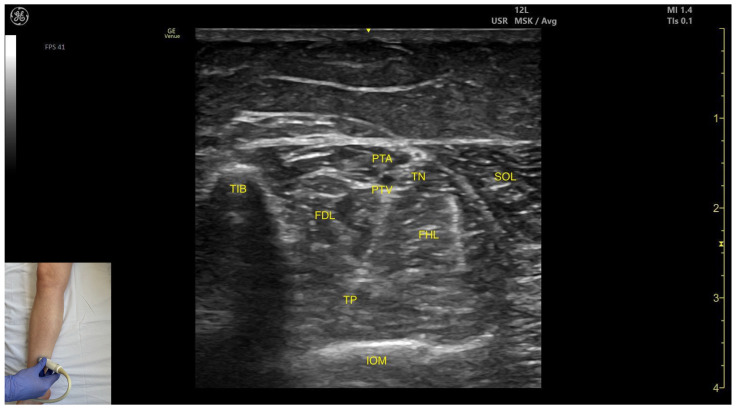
Ultrasound anatomy of the tibialis posterior—medial window: TIB—tibia; FDL—flexor digitorum longus; TP—tibialis posterior; PTA—posterior tibial artery; PTV—posterior tibial vein; TN—tibial nerve; FHL—flexor hallucis longus; IOM—interosseous membrane; SOL—soleus.
